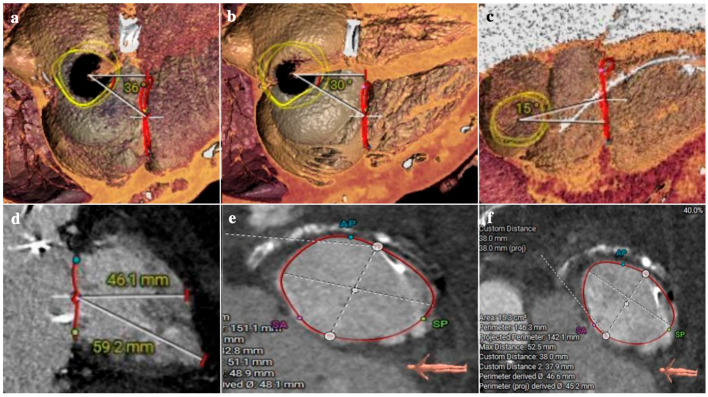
Cardiac CT screening of candidate patient for Cardiovalve implantation: different take-off of the IVC and relationship between IVC and tricuspid annulus (a–c). Measurement of the distance between the tricuspid annular plane and the free-wall of the RV (d); systolic and diastolic dimensions of the annulus together with the evaluation of minimal and maximum diameter (e,f). CT: computed tomography; IVC: inferior vena cava; RV: right ventricle.
